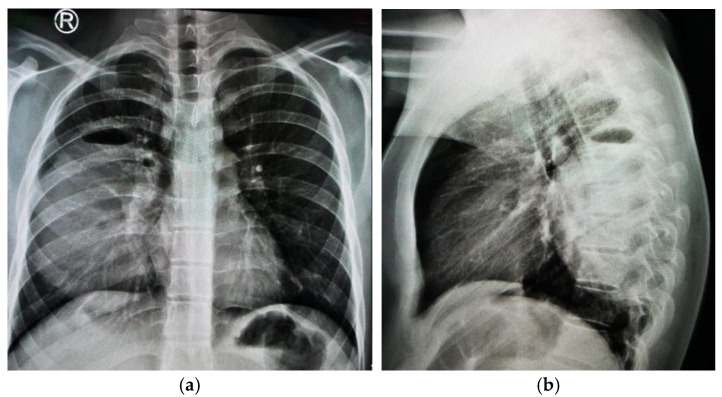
Seventeen-year-old male adolescent with pulmonary abscess: enlargement of oval-shaped mass, measuring approximately 15 cm × 9 cm × 12 cm, characterized by thick walls and containing two air–fluid levels observed in upper third of mass, with increased radiopaque component noted; (a) posteroanterior chest X-ray; (b) lateral chest X-ray.
